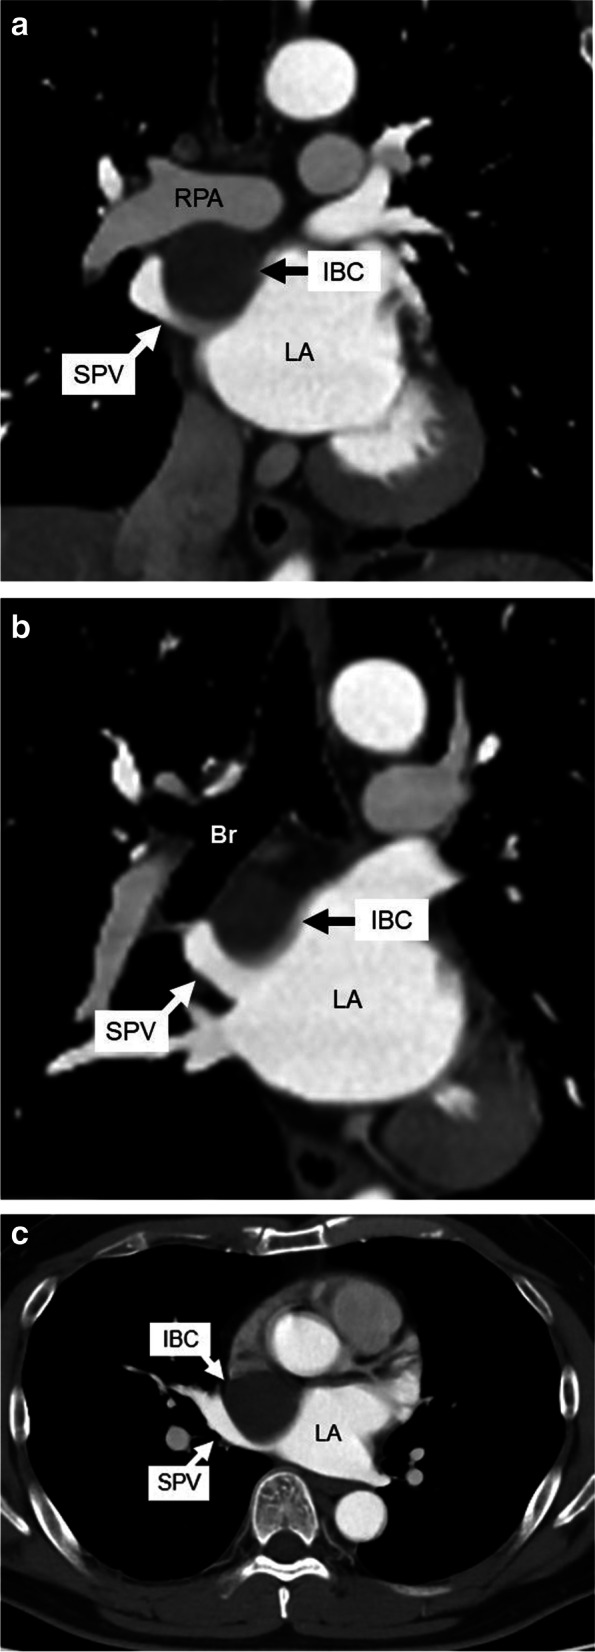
Coronal and axial view of preoperative chest CT. a A 42 × 35 × 32-mm cystic mass at the intrapericardium located under the RPA and compressing the SPV and LA. b The cystic mass attached to the right bronchus and compressing the SPV and LA. c The cystic mass compressing the SPV and LA
